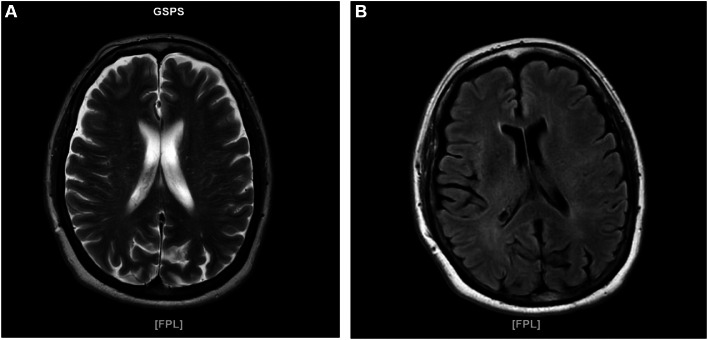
Non contrast MRI in T2W (A) and T2-FLAIR (B) showed no sign of bleeding to exclude the thrombolysis contraindication.
